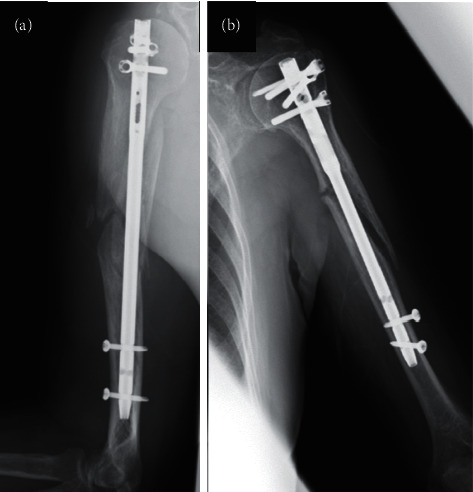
X-ray radiographs showing delayed bone union. A 70-year-old man sustained a humeral shaft fracture (AO type A1) and underwent an intramedullary nailing eight days after injury. A plain radiograph performed 26 weeks postoperation showed a visible fracture line (a). An 80-year-old woman sustained a humeral shaft fracture (AO type A2) and underwent an intramedullary nailing 11 days after injury. A plain radiograph performed 30 weeks postoperation revealed a visible fracture line and loosening distal screws (b).
